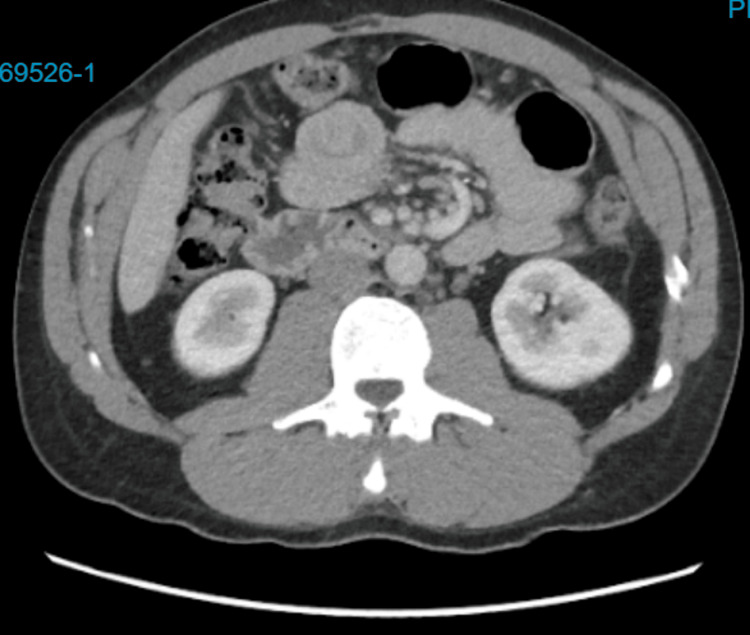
CT scan of the abdomen demonstrating a different area of intussusception with the target sign and proximal thickened jejunal bowel loops.
